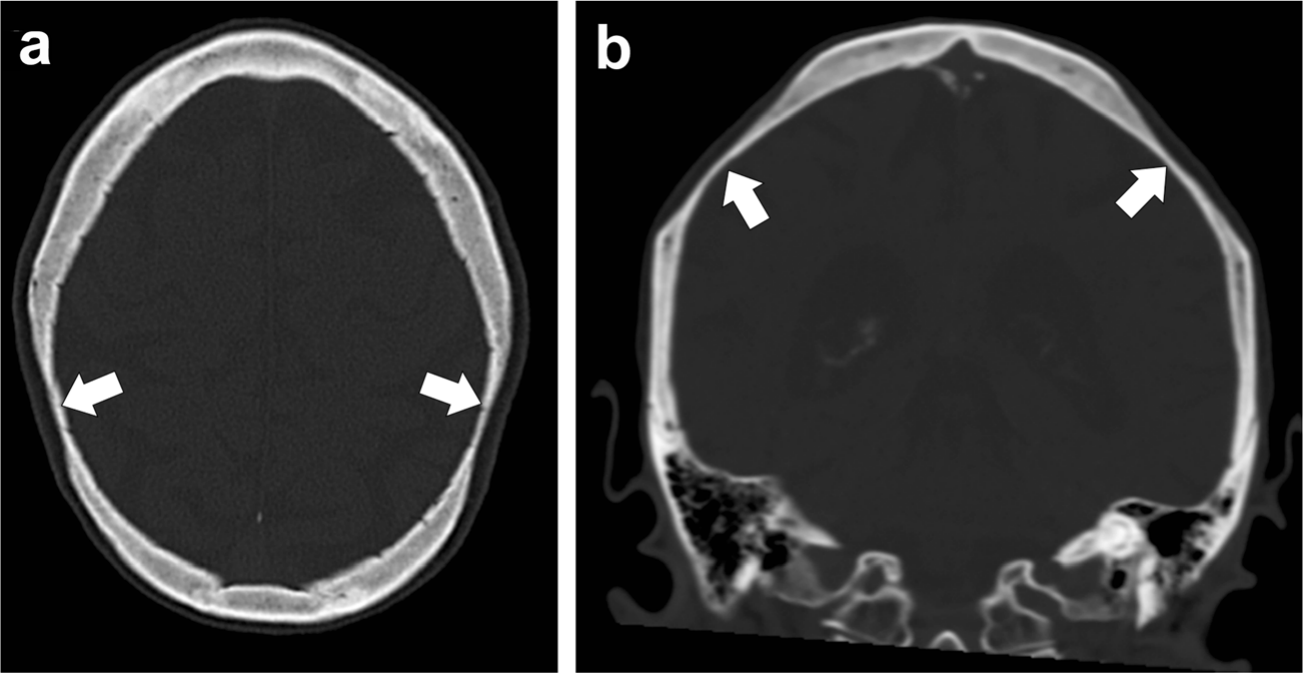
Parietal thinning. Axial (a) and coronal (b) head CT images shows thinning of the parietal bones (*thick arrows*)
